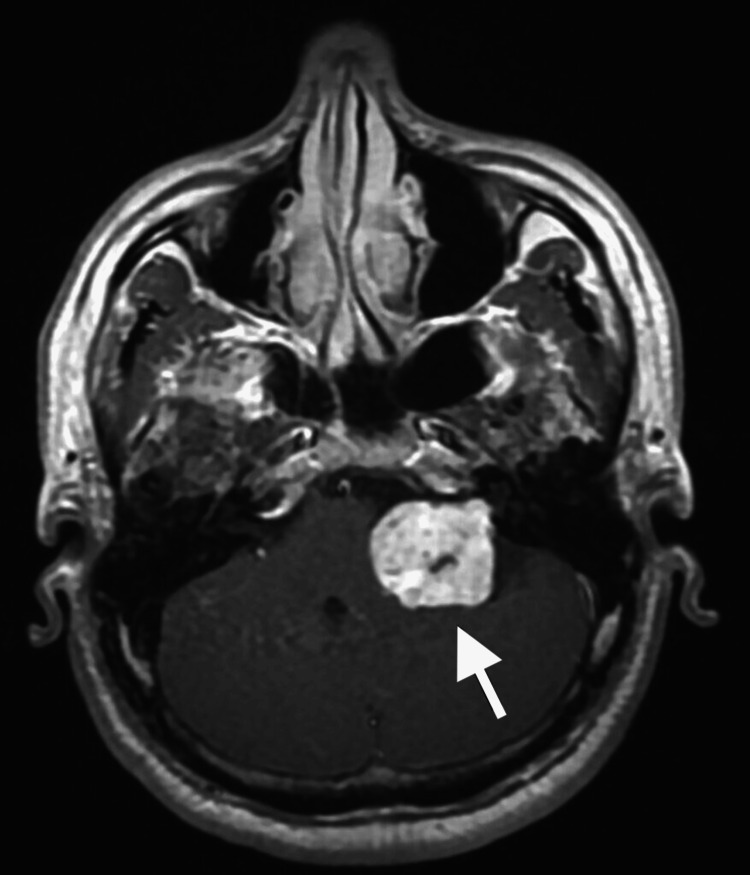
Preoperative MRI (T1-weighted with contrast) demonstrating a left-sided vestibular schwannoma measuring 38.2 × 31.3 mm (white arrow)
